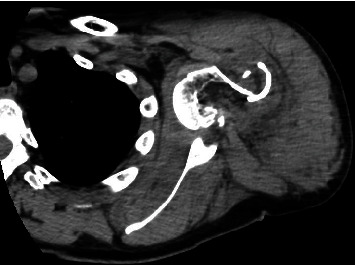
Axial CT scan, left shoulder (case 2). Comminuted fracture of the proximal humerus with complete anterior dislocation of the glenohumeral joint.
